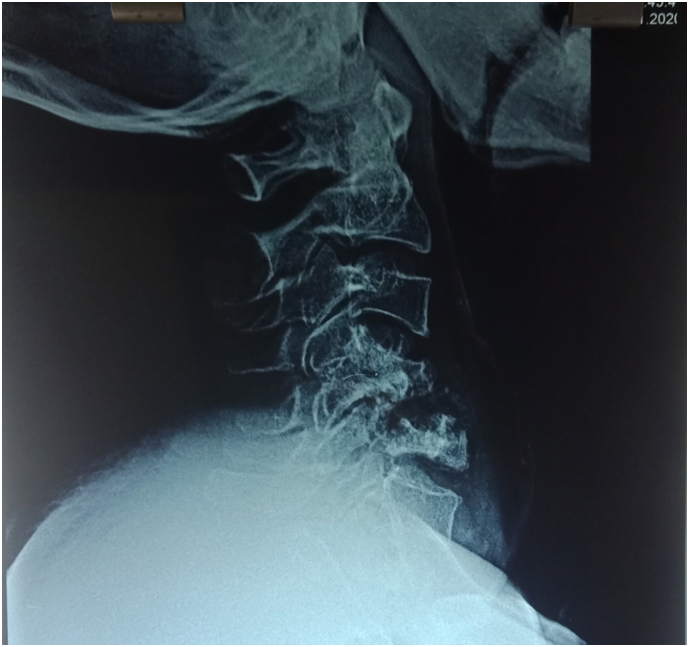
Pre-operative lateral cervical X-ray.
